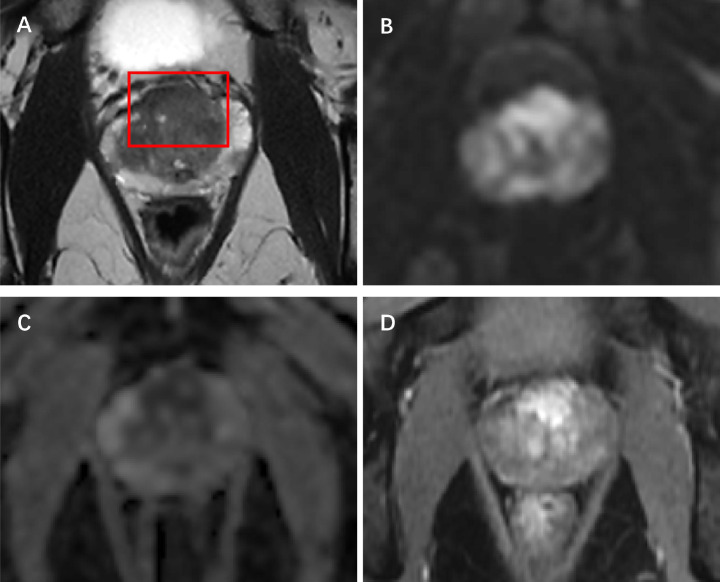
A 66-year-old man with PSA 11.6 ng/ml, prostate MRI revealed a lesion in the transition zone posterior to the anterior fibromuscular stroma (red box) on (a) axial T2-weighted image, (b) axial diffusion-weighted image with b value of 2000 mm2/sec, (c) axial apparent diffusion coefficient map, and (d) axial dynamic contrast-enhanced image in prostate MRI. Gleason score 4 + 3 prostate cancer confirmed after radical prostatectomy. The PI-RADS scores assigned by the four readers were 4, 3, 2, and 2 in bpMRI respectively, as well as 4, 4, 2, and 2 in mpMRI respectively. mpMRI, multiparametric MRI; bpMRI, biparametric MRI; PSA, prostate-specific antigen; PI-RADS, Prostate Imaging Reporting and Data System.
